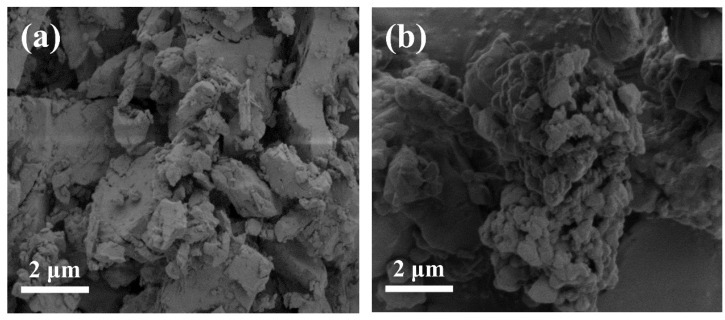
SEM images of (a) the original CaCO_3_ and (b) CaCO_3_ modified with KH550 and HY311 coupling agents combined with ultrasound treatment.
